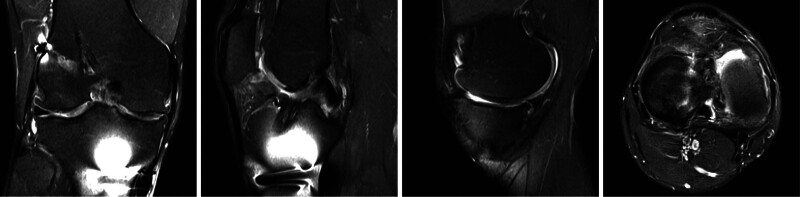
Preoperative coronal, sagittal, and axial magnetic resonance imaging images. The images are of the right knee of a 36-year-old male patient undergoing second revision anterior cruciate ligament reconstruction. The images show anterior cruciate ligament graft injury, medial meniscus injury, and medial articular cartilage injury.
